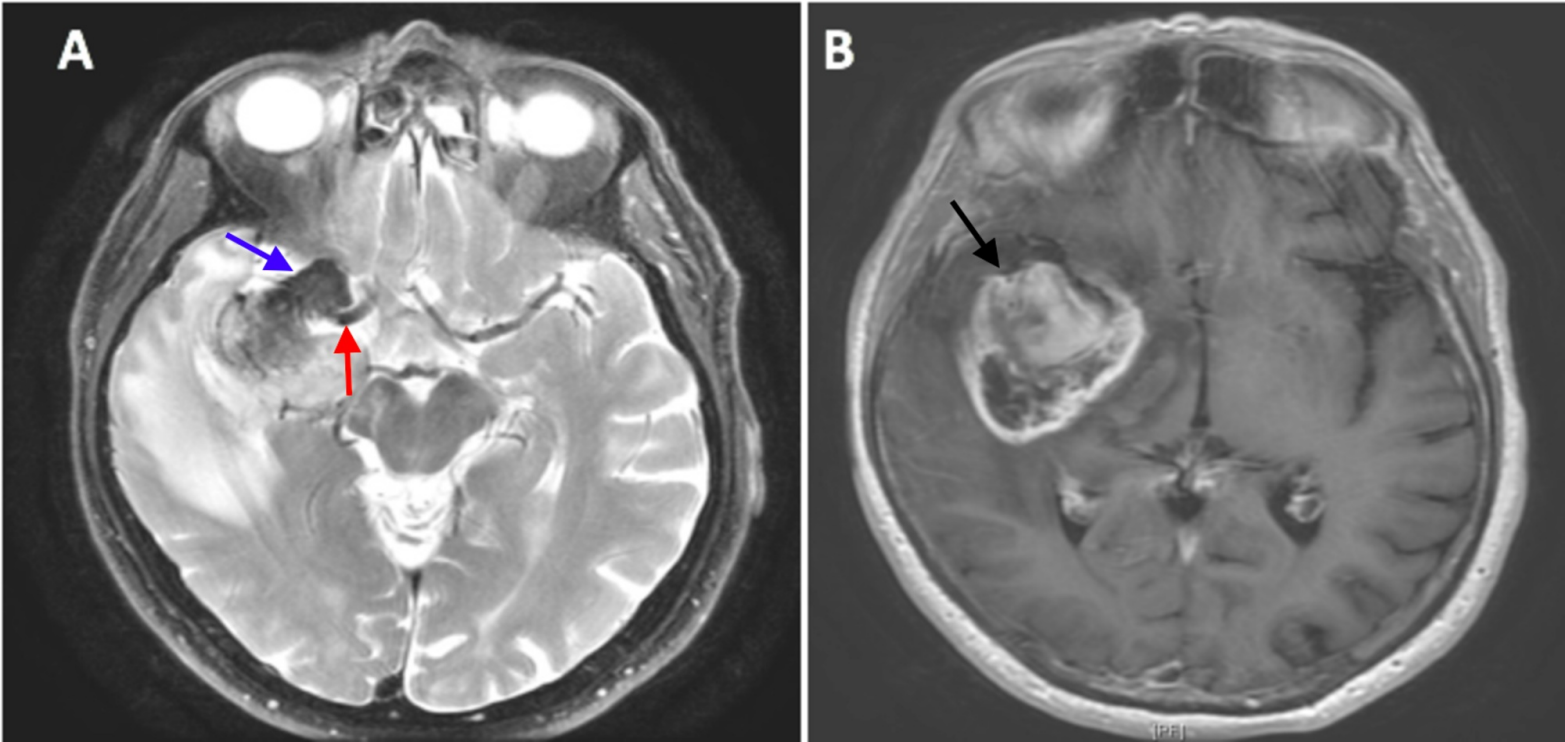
Magnetic resonance imaging (MRI) of patient's brain. (A) Axial T2 MRI with extramedullary lesion (blue arrow) adjacent to middle cerebral artery (red arrow); (B) T1 post-gad MRI with surround enhancing lesion (black arrow) with central necrosis consistent with Glioblastoma Multiforme.
